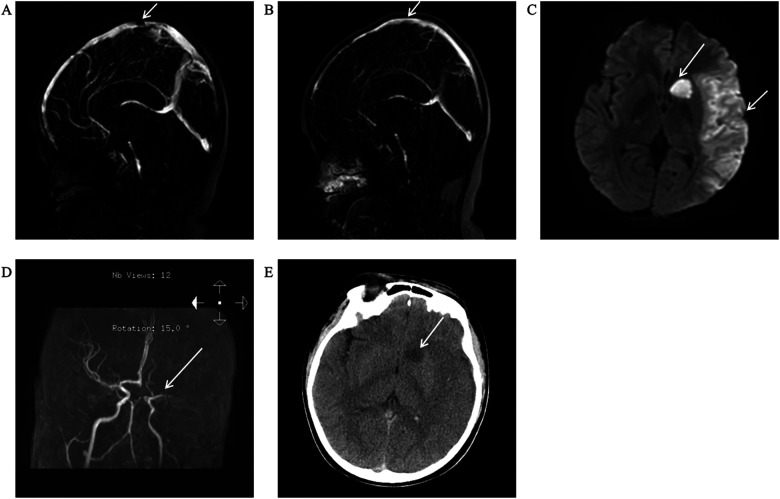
Representative neuroimaging findings of cerebrovascular thrombosis in pediatric acute lymphoblastic leukemia. (A) Magnetic resonance venography (MRV) demonstrating superior sagittal sinus thrombosis at symptom onset in patient CVST-5. (B) Follow-up MRV showing partial recanalization on subsequent imaging. (C) Diffusion-weighted imaging (DWI) revealing acute infarction in the left middle cerebral artery territory in patient AIS-1. (D) Magnetic resonance angiography (MRA) demonstrating occlusion of the left middle cerebral artery (M1 segment). (E) Post-thrombectomy computed tomography (CT) confirming vascular recanalization without hemorrhagic transformation.
